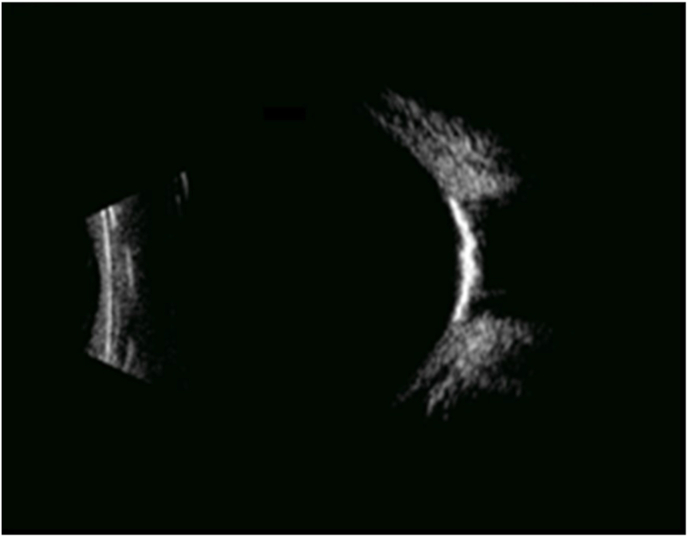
B-scan ultrasonography showed a sharply demarcated hyperechoic choroidal lesion with posterior shadowing.
